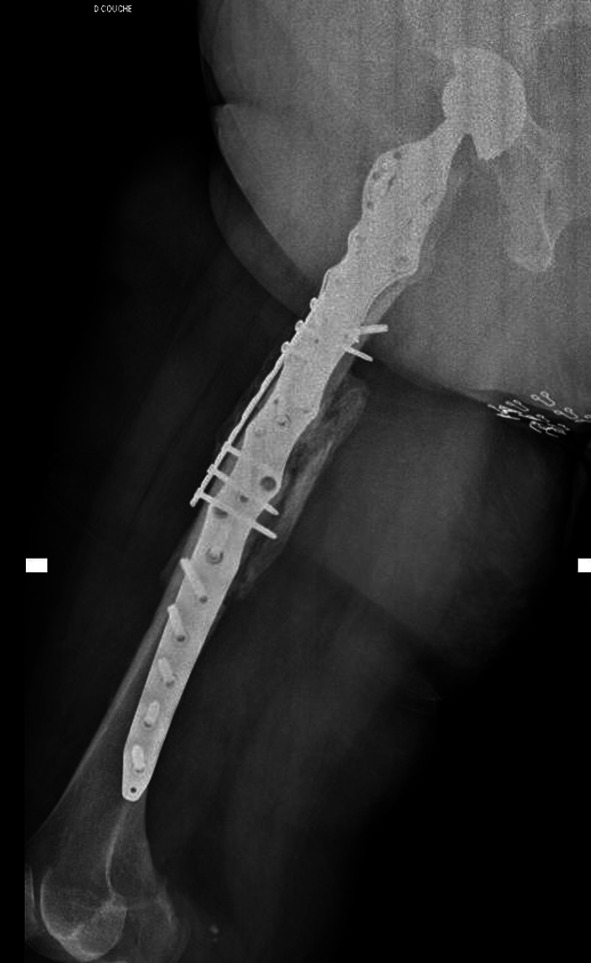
Posterior union on the lateral X-ray.
